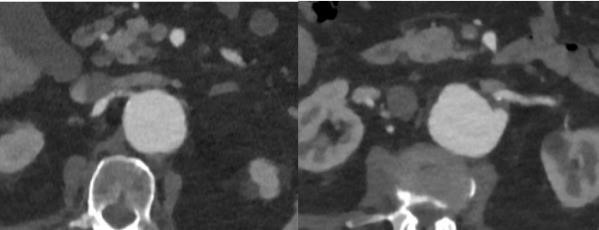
Preoperative CT demonstrating a juxtarenal 5.5 cm
